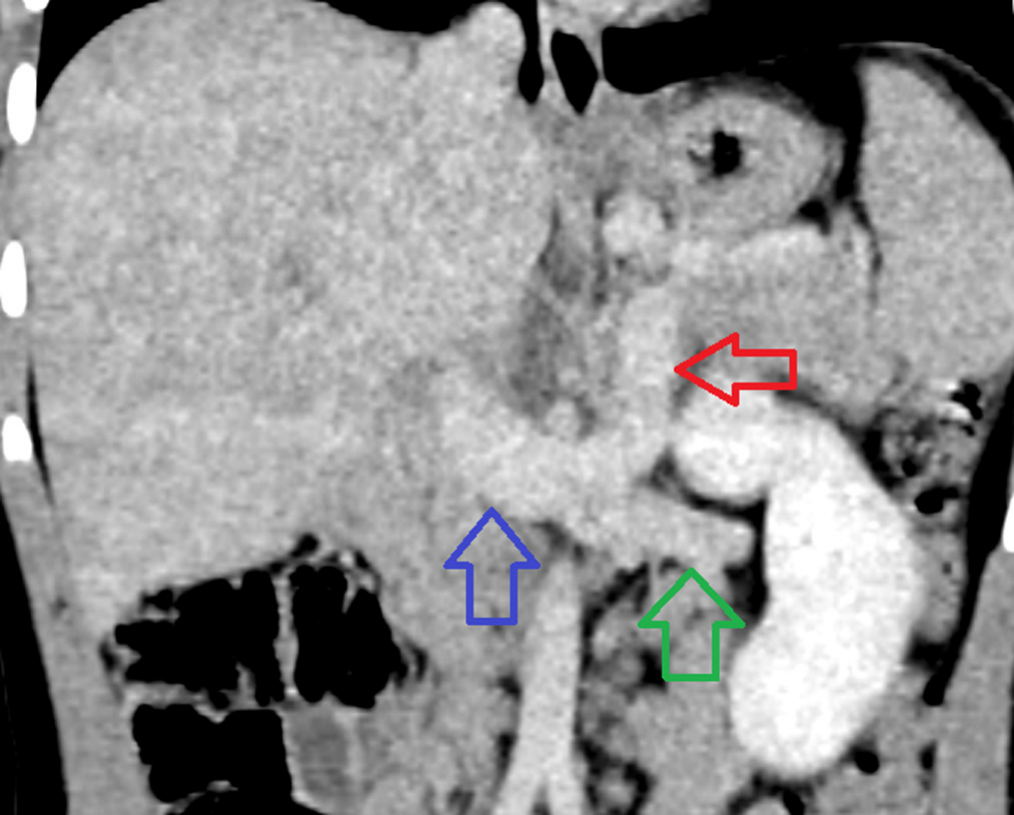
Coronal image of a CT of the abdomen and pelvis with IV contrast in the venous phase of imaging (red arrow). There is a portosystemic shunt that opacifies during the during phase of imaging. This vessel courses inferiorly and enters into the confluence of the left renal vein (green arrow) and IVC (blue arrow). CT = computed tomography; IV = intravenous; IVC = inferior vena cava.
